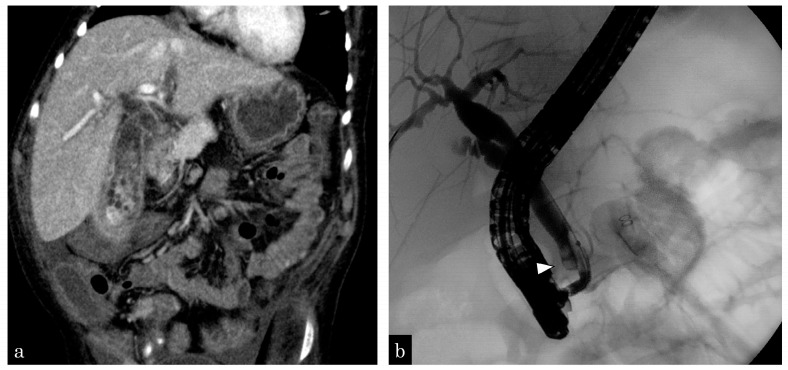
An 8-year-old boy complained of gallbladder and common bile duct stones. (a) Enhanced computed tomography (CT) revealing multiple small gallstones and gallbladder swelling. (b) The patient has developed septic shock due to cholangitis. Emergency gallbladder drainage is performed, and a bile stone impacting the distal common bile duct (white arrowhead) is observed. A 4-Fr ENBD tube is inserted.
